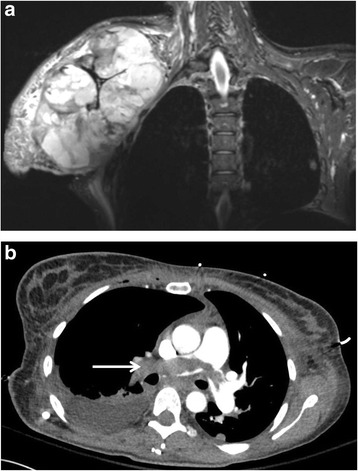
Primary tumor and pulmonary embolism. a Coronal chest MRI of a 36-year-old female with a recurrent right upper extremity myxofibrosarcoma. b Chest computed tomography of pulmonary artery saddle embolism (*arrow*)
